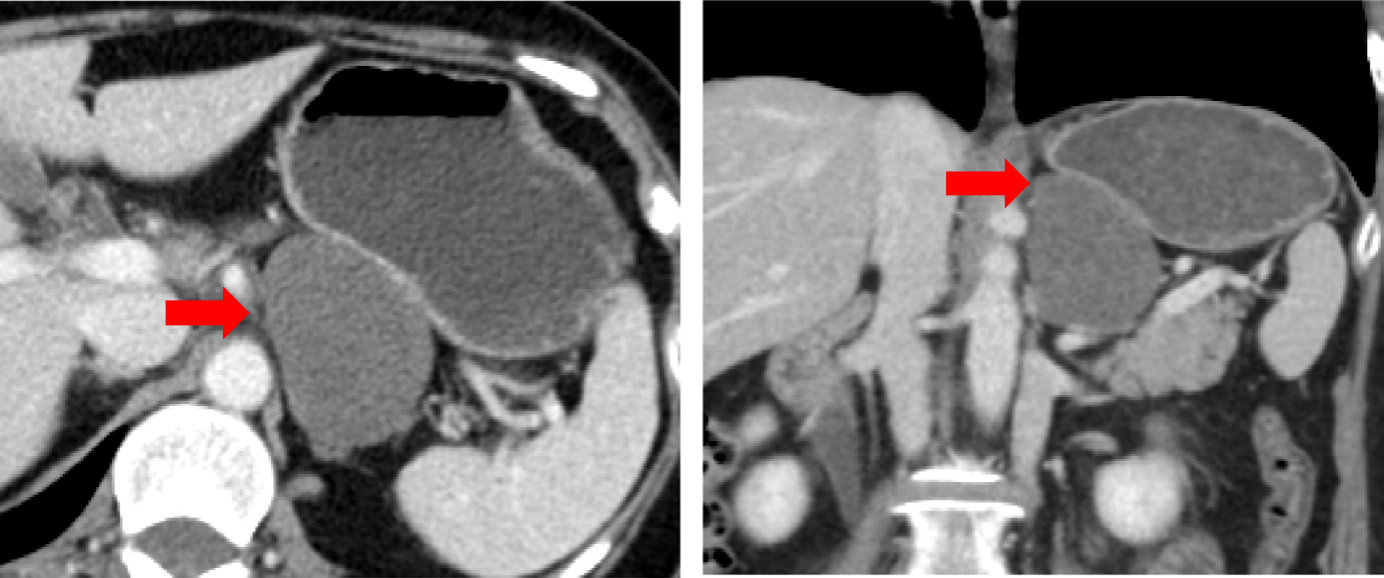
Abdominal contrast‐enhanced computed tomography images. A 56.7 × 37.9 × 54.7 mm between the posterior wall of the gastric corpus and the right margin of the abdominal aorta (red arrows)
